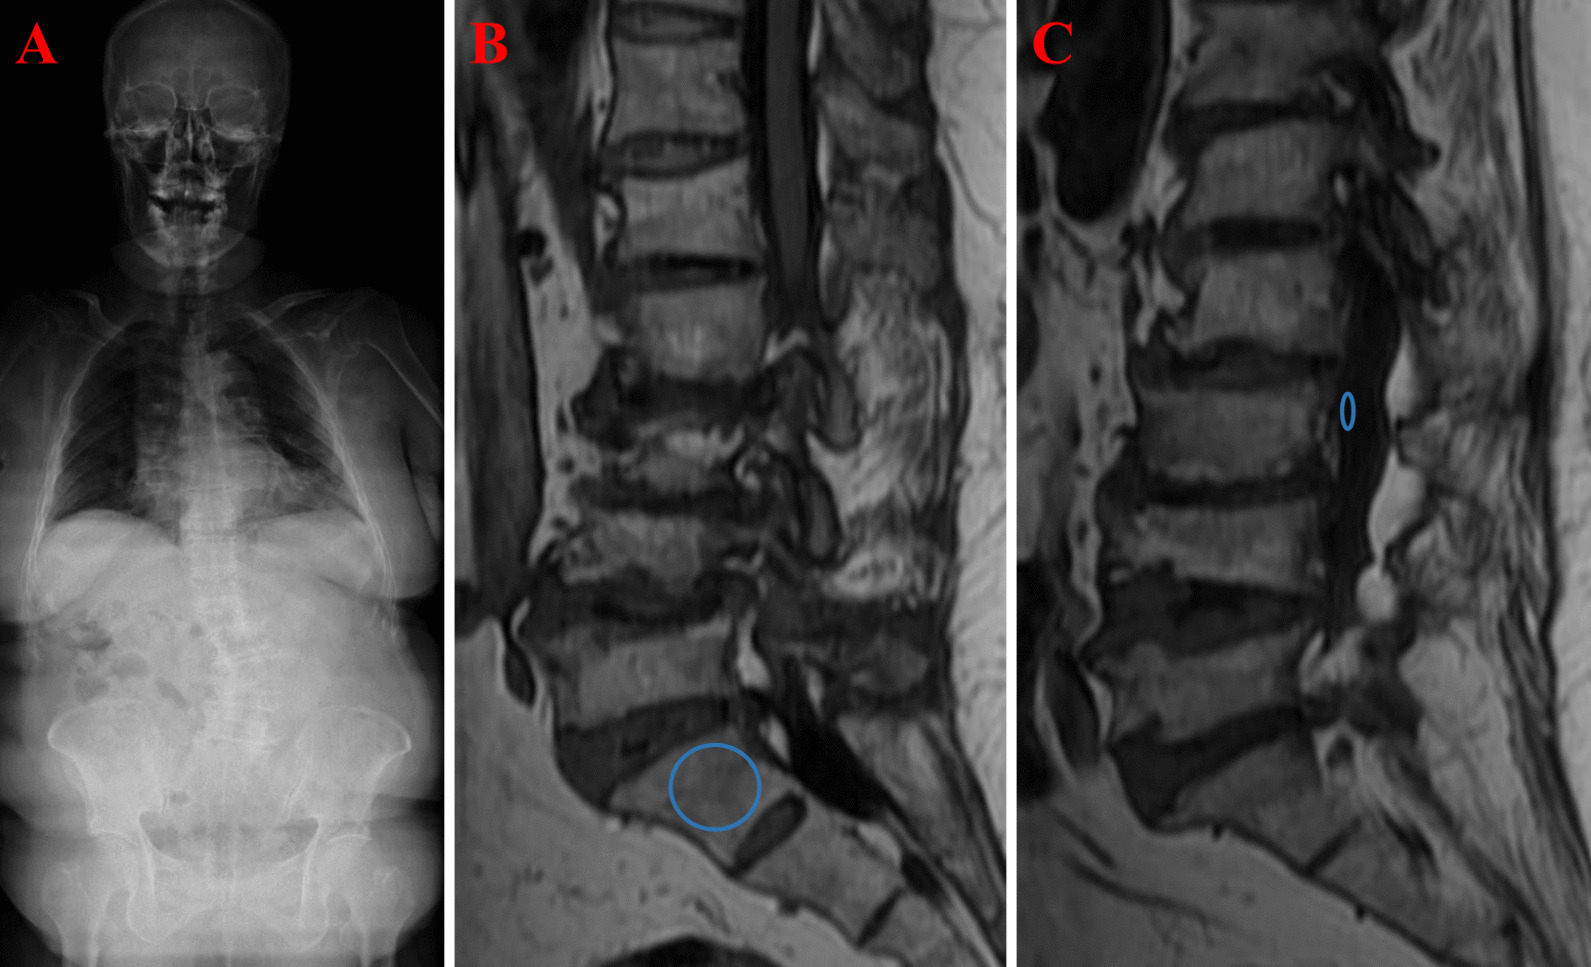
A A typical standing full-length posteroanterior plain radiography of DLS clearly showed the structural lumbar curve (B, C). Sagittal non-contrast-enhanced T1-weighted MRI of the lumbar spine detailing the SI of the ROI (circles) used to calculate the S1 VBQ score
